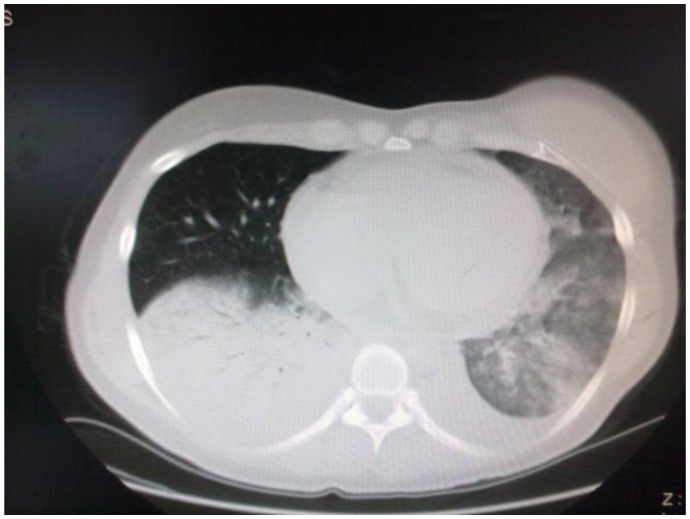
CT Scan before posaconazole treatment.
